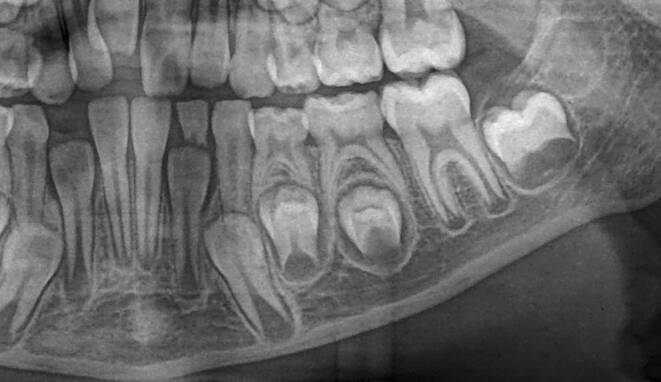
Orthopantomogram section of the lower left quadrant with different Demirjian stages (31: H, 32: F, 33: F, 34: D, 35: C, 36: G, 37: C) Orthopantomogramm-Ausschnitt des linken unteren Quadranten mit verschiedenen Demirjian-Stadien (31: H, 32: F, 33: F, 34: D, 35: C, 36: G, 37: C)
